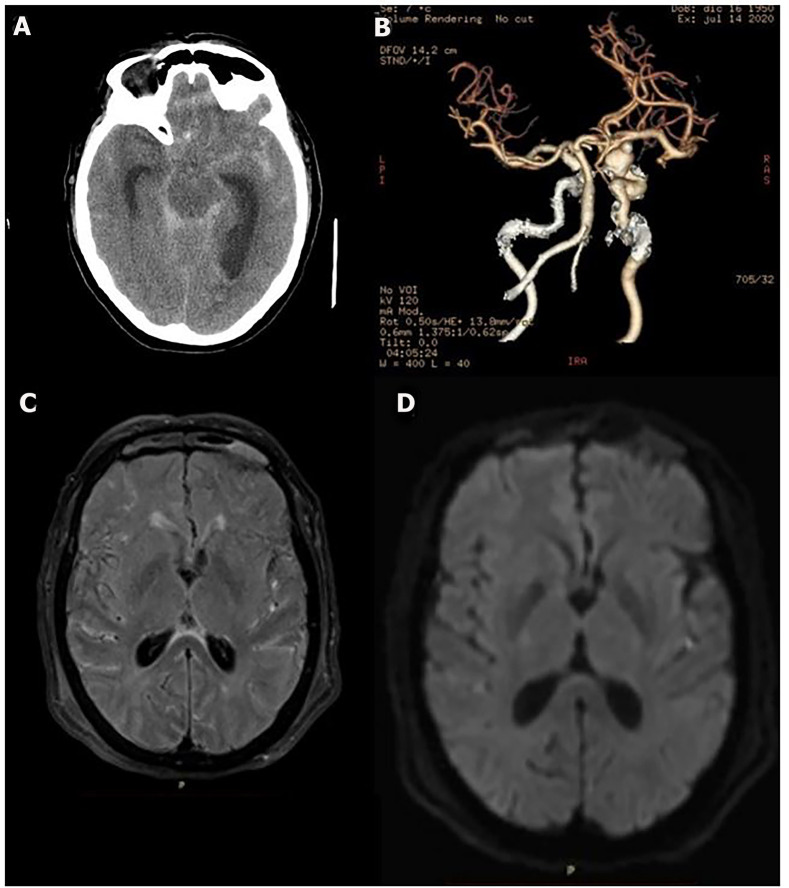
Neuroimaging studies of the case. (A) Head computed tomography scan showing diffuse subarachnoid hemorrhage with intraventricular hemorrhage and hydrocephalus; (B) computed tomography angiogram showing a right supraclinoid internal carotid artery aneurysm; (C) FLAIR magnetic resonance imaging and (D) diffusion magnetic resonance imaging show no abnormalities.
